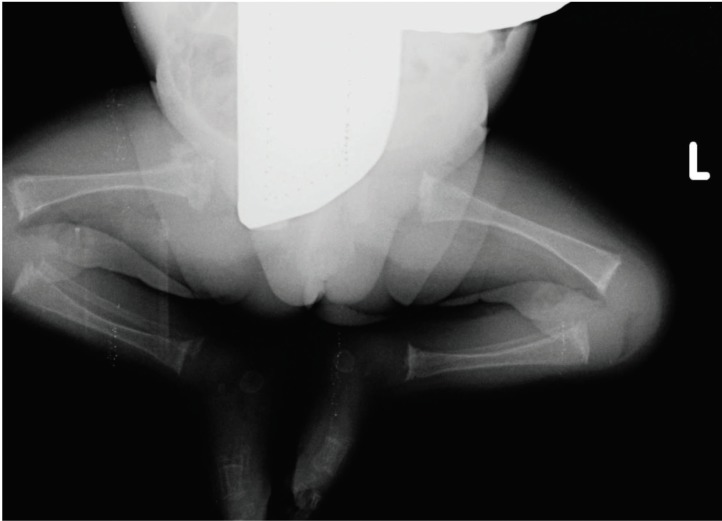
X-Ray (bilateral lower limbs) showing generalized osteopenia.
